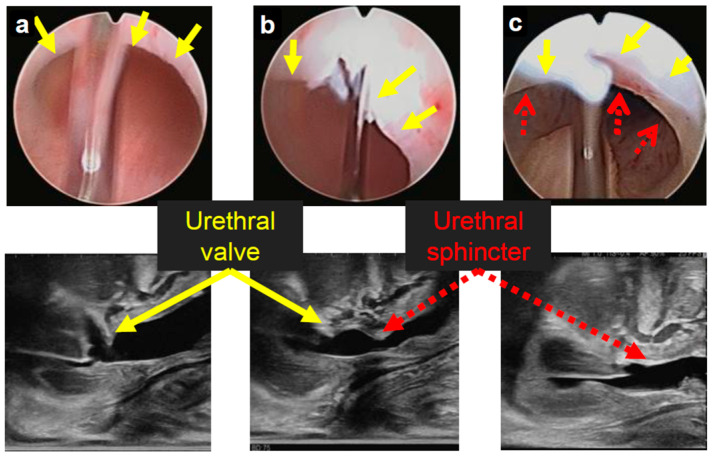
Comparison of urethral ultrasound and urethroscopic findings in the same patient. (a) PUV is observed on the ventral side (solid-line arrow). (b) Initial incision of PUV. Although the urethral sphincter is not visible in the urethroscopic image, it is shown on ultrasound image in close proximity to the PUV (dashed-line arrow). (c) Both the urethroscopic and ultrasound images confirm the presence of the urethral sphincter. PUV: posterior urethral valves.
